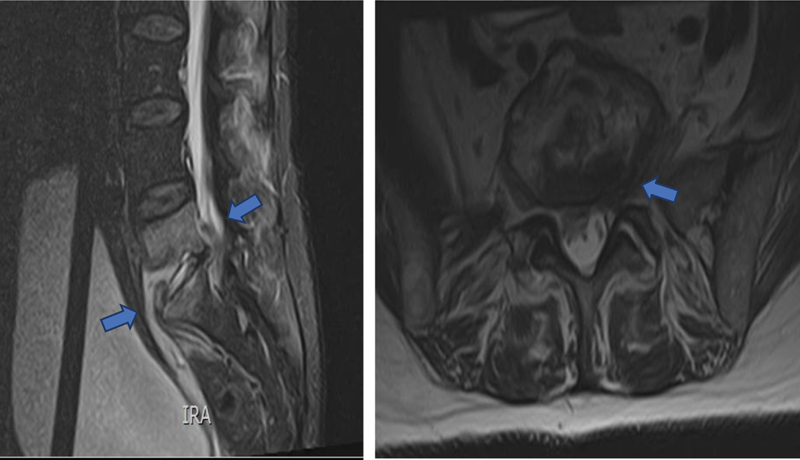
Magnetic resonance imaging before treatment – Accentuated edema of the somatic L5 and S1 sponge with erosive anomalies in the vertebral plates, suggestive of spondylodiscitis. Associated phlegmon with anterior extension to the sacrum and to the posterior peridural region.
